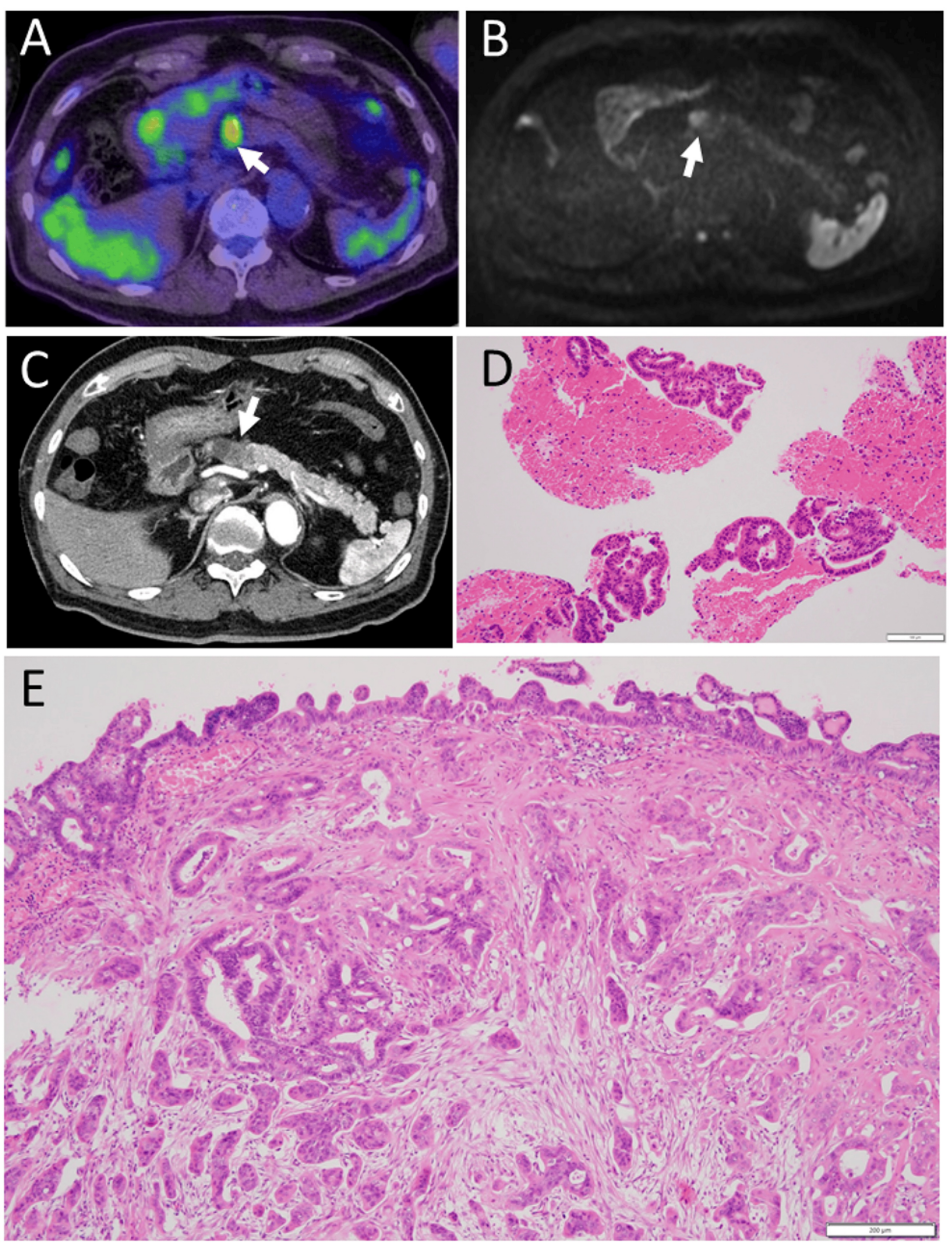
Pancreatic lesion. Positron emission tomography, one year later at 77 years old, showing abnormal uptake (arrow) in pancreatic body (A). Magnetic resonance imaging just before trans-gastric pancreatic biopsy, showing high signal of pancreatic head to body (arrow) in diffusion-weighted image (B). Computed tomography scan with enhancement at the age of 78 years just before pancreaticoduodenectomy, showing a low-enhanced mass with a cyst (arrow) in the pancreatic head to body (C). Trans-gastric fine needle pancreatic biopsy (D), showing adenocarcinoma. Subtotal stomach-preserving pancreaticoduodenectomy with jejunostomy (E), showing intraductal papillary mucinous carcinoma with interstitial invasion. White scale bar =100 µm in D, 200 µm in E.
